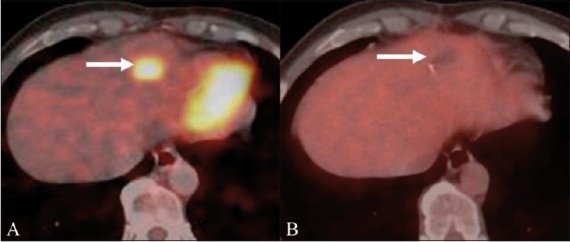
FDG PET / CT evaluation of a solitary hepatic metastasis in an operated case of colon cancer, treated with radiofrequency ablation (RFA). Pre-RFA fused PET / CT (A) image shows an FDG-avid metastasis in the left lobe of the liver (arrow). Immediate post-RFA fused PET / CT (B) image shows complete photopenia at the ablated site (arrow), suggesting complete ablation
